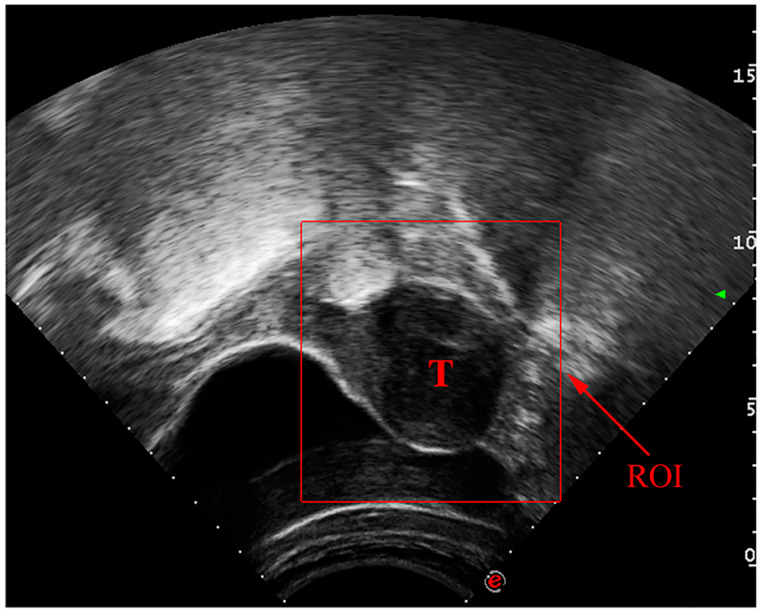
Ultrasound image of benign tumor of uterine fibroids.
